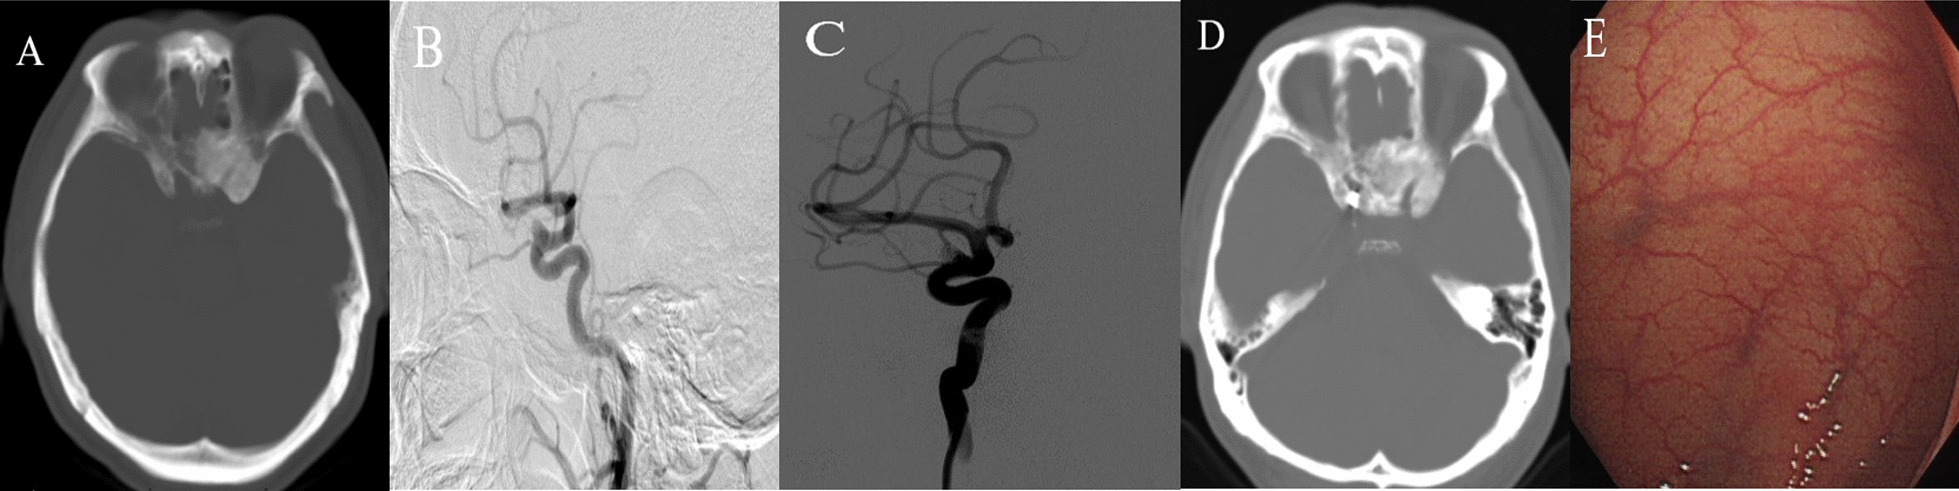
CT showing FD of the left sphenoid bone (A) and DSA showing an aneurysm in the right internal carotid artery (C6) (B). Stent assisted coil embolization was done and there was no filling of aneurysm (C). After 6 years, his FD showed no sign of progression (D). At the same time, the patient was diagnosed with colonic vascular malformation by colonoscopy (E)
